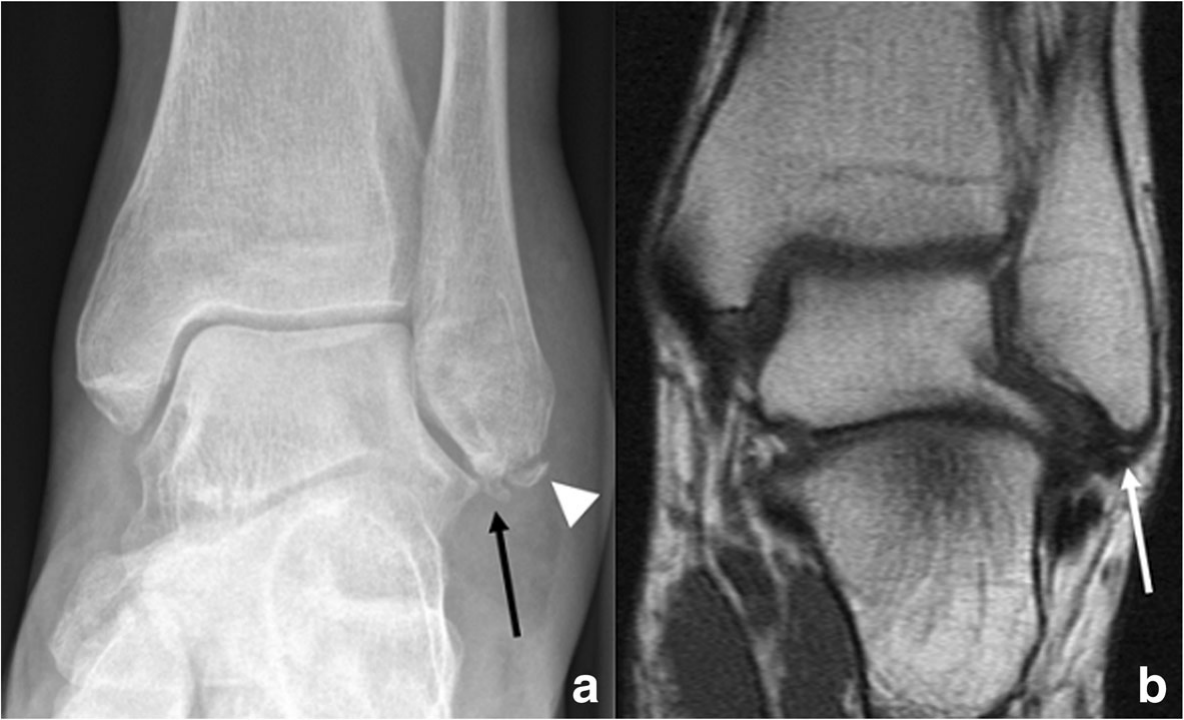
Differential diagnosis of os fibulare. a AP radiograph in a 67-year-old woman, history of inversion injury. There is a small avulsion of the tip of the malleolus, in keeping with injury to the lateral collateral ligament (arrow head). Medial to this, there is a small ossified body (black arrow). This has rounded margins. Findings are compared with previous MR, performed 10 years before. b Coronal FSE T1 demonstrates a small ossified body was present already (white arrow). MR was performed with the suspicion of talonavicular osteoarthritis at the time. A small rounded structure could have already represented a sequel of avulsion injury but was described as an ossicle in the absence of acute trauma
